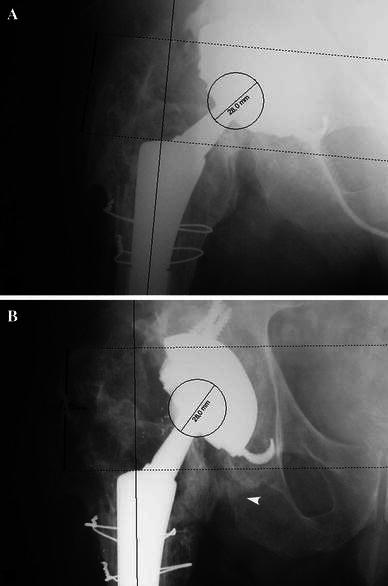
Stem subsidence was measured by processing immediate postoperative (a) and 3-month (b) follow-up anteroposterior radiographs via Roman v1.7 software (Roman free to share software version V1.70; Robert Jones and Agnes Hunt Orthopaedic Hospital, Oswestry, UK; http://www.Keele.ac.uk/depts./rjah/), as a change in the vertical distance from the proximal tip of the greater trochanter to the shoulder of the stem. Ectopic bone formation at the lesser trochanter area (*white arrowhead*) is noted 3 months postoperatively (b)
